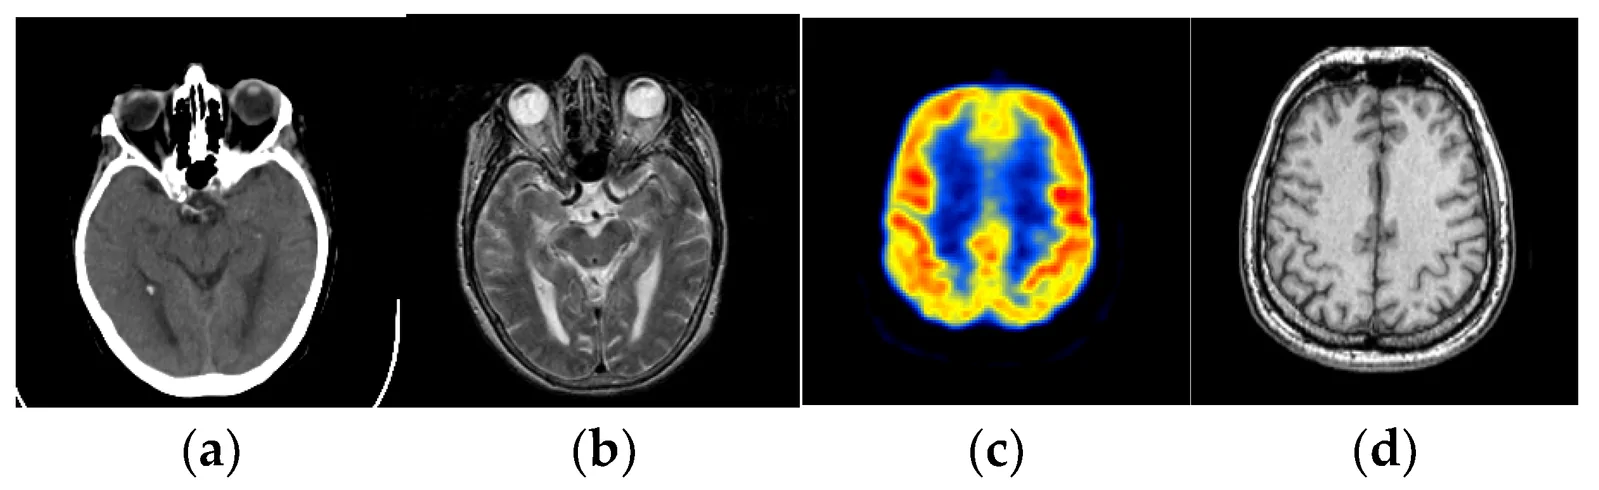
The original image for (a) CT; (b) MRI; (c) PET; (d) MRI, where (a), (b), and (d) are grayscale intensity images where pixel brightness indicates the X-ray attenuation (CT) and MR signal intensity (MRI), while (c) is a PET image in which warm hues indicate high and cool hues indicate low radiotracer uptake.
